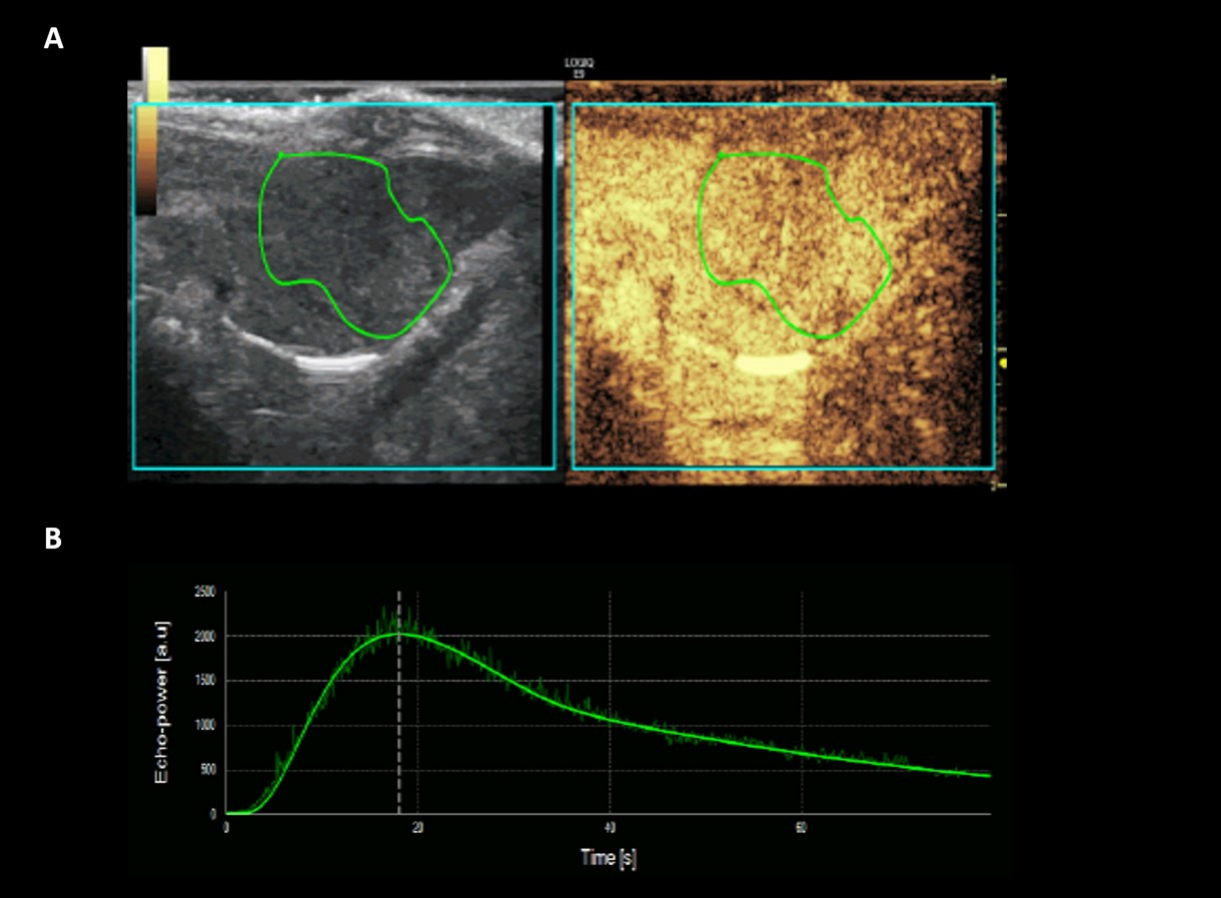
Representative case of CEUS image analysis using perfusion analysis software (VueBox ®), ROI manually drawn over tumor (A), and corresponding time-intensity curve profile (B). Note–CEUS: contrast enhanced ultrasound examination. ROI: region of interest.
